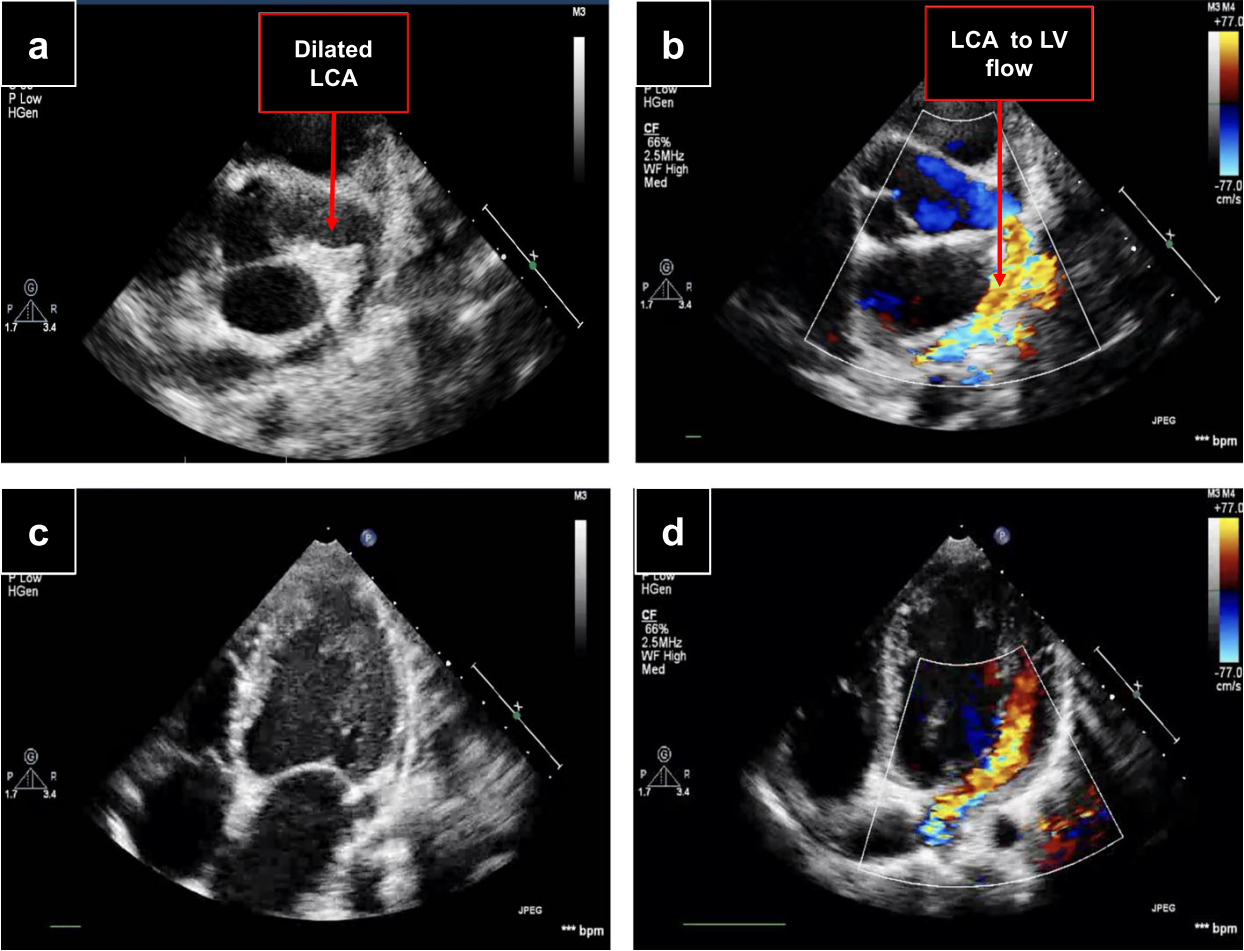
Echocardiogram images of case 2. Parasternal short-axis views showing a dilated LCA origin (a) with color flow Doppler (b). Apical four-chamber views showing dilated LV (c) and color flow Doppler showing fistulous flow entering the dilated LV (d). *LCA* left coronary artery, *LV* left ventricle
